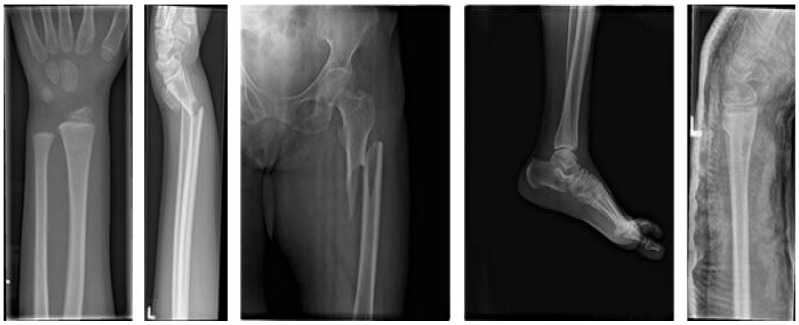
Sample Images from the X-ray Fracture Dataset.
